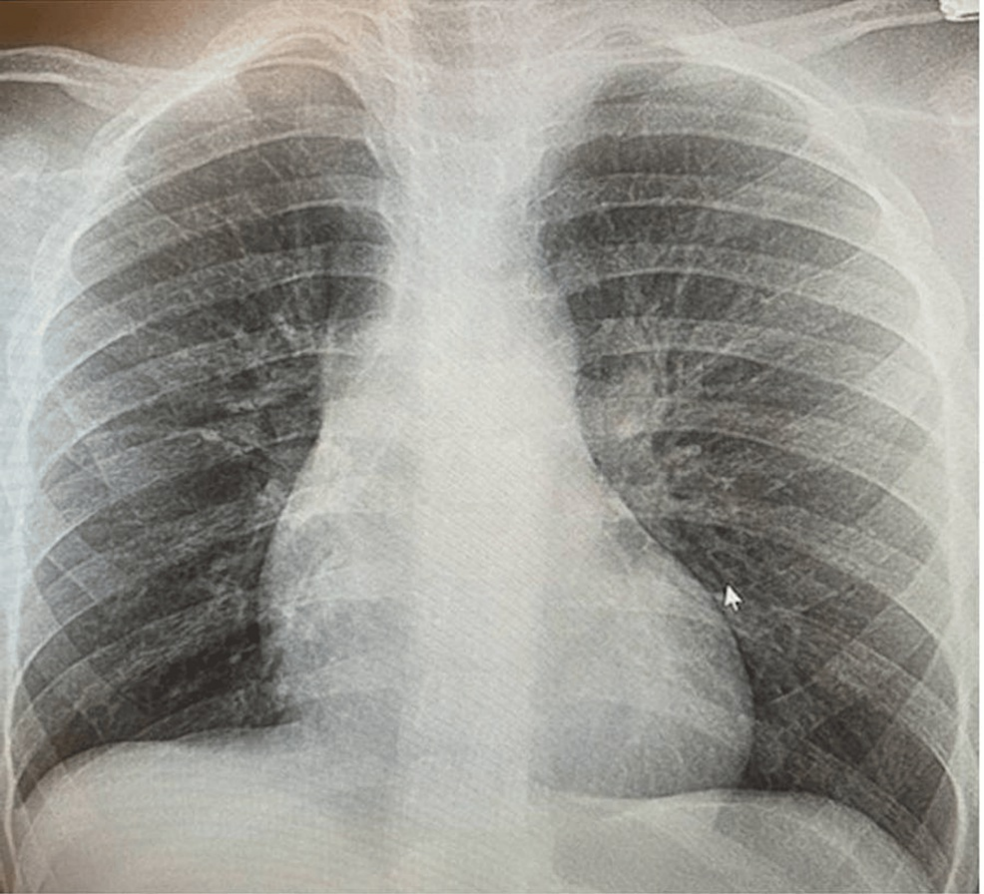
Chest X-ray from 2020 displaying no confluent infiltrate or effusion.
